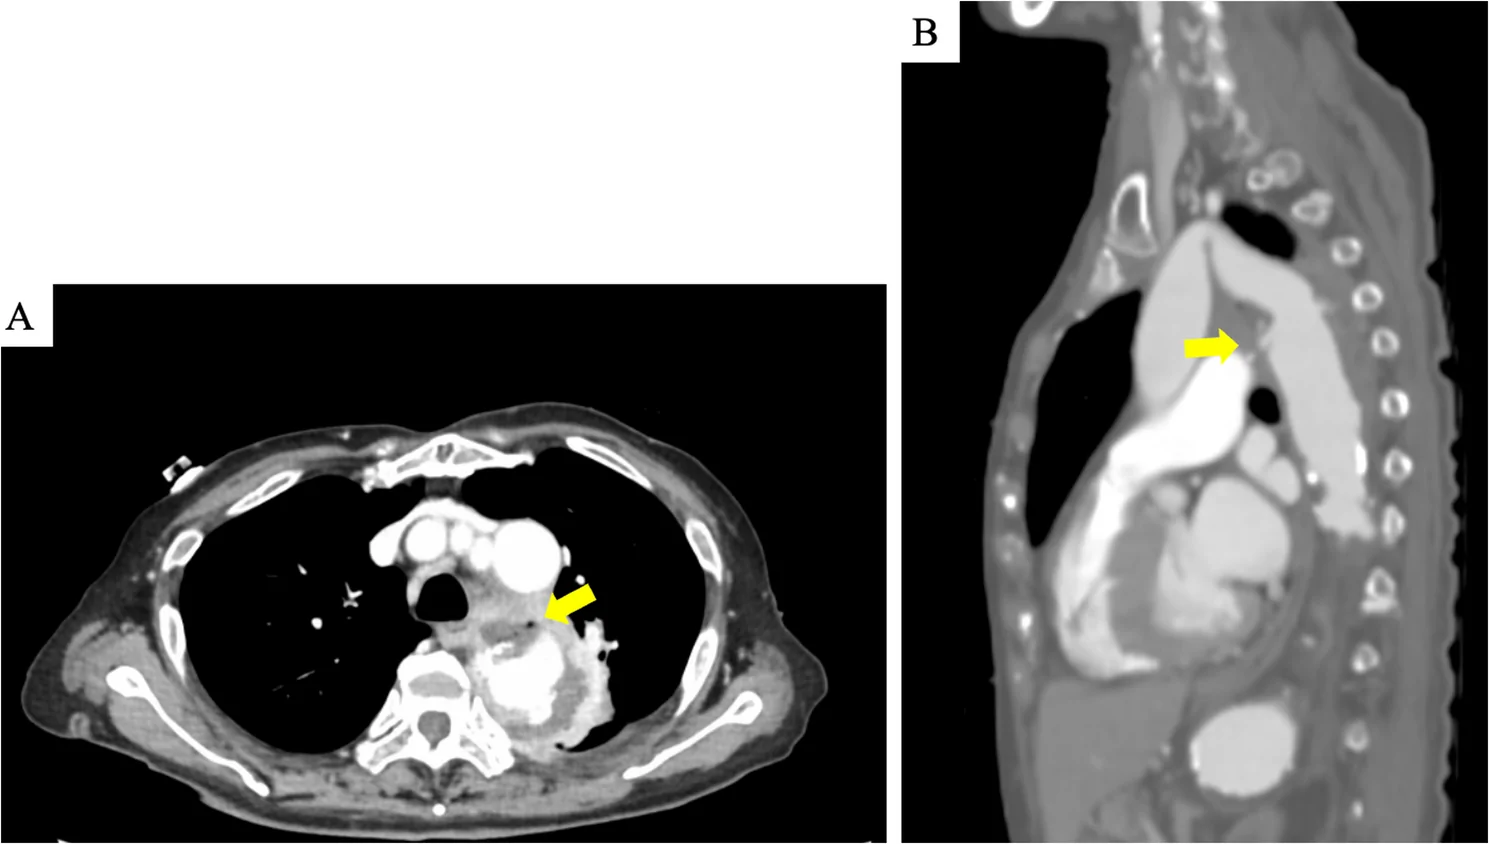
Contrast-enhanced CT findings on admission. A Axial contrast-enhanced CT image demonstrating peri-aortic gas and fluid collection with obliteration of the intervening fat plane between the oesophagus and prosthetic aortic graft (arrow), strongly suggestive of secondary aorto-oesophageal fistula. B Sagittal contrast-enhanced CT image showing the anastomotic site (arrow) adjacent to the suspected fistulous region.
